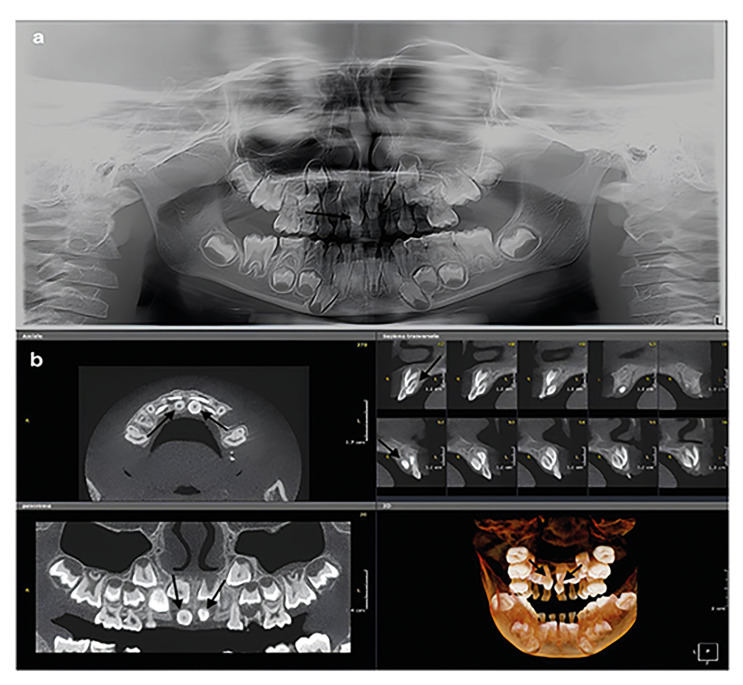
Double mesiodens in a 5-year-old girl. (a) Panoramic radiograph. Two supernumerary teeth (arrows) are appreciable between 11 and 21 above, and 51 and 61 below. (b) CBCT. The two mesiodens (arrows) are vertical, palatal oriented, had a conoid shape and are contiguous with the nasopalatine canal.
